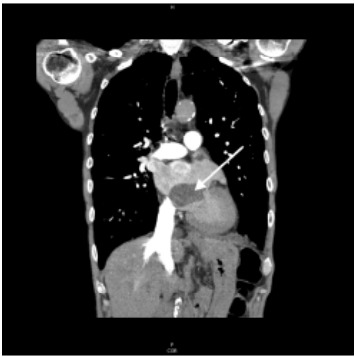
Computed tomography axial view demonstrating a 3 x 3 x 5 centimeter left atrial mass (arrow) later confirmed to represent an atrial myxoma.
